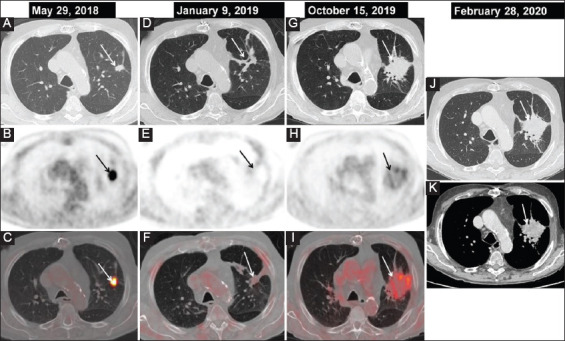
A 83-year-old male, ex-smoker, with heart transplant for ischemic cardiomyopathy with severe heart failure in 2000. Severe COPD. Stage I squamous cell carcinoma of the lung cells in the upper left lobe. 18F-fluorodeoxyglucose (^18^F-FDG) positron emission tomography/computed tomography (PET/CT) images before treatment with stereotactic body radiation therapy (SBRT) showed a solid and spiculated nodule in the upper left lobe with FDG capture and SUVmax 13.3 (A, B, and C) (arrows). Three months after SBRT the PET/CT shows changes consistent with patchy consolidation pneumonitis, associated with volume loss and decrease of the SUVmax to 2.3 (arrows). ^18^F-FDG PET/CT 12 months after SBRT (G, H, and I) shows a modification in lung changes, with rounded “mass-like” morphology and peripheral FDG uptake, with the SUV maximum of 3.5 (arrows). Image results are stable on a CT scan performed 16 months after SBRT (J and K) (arrows).
